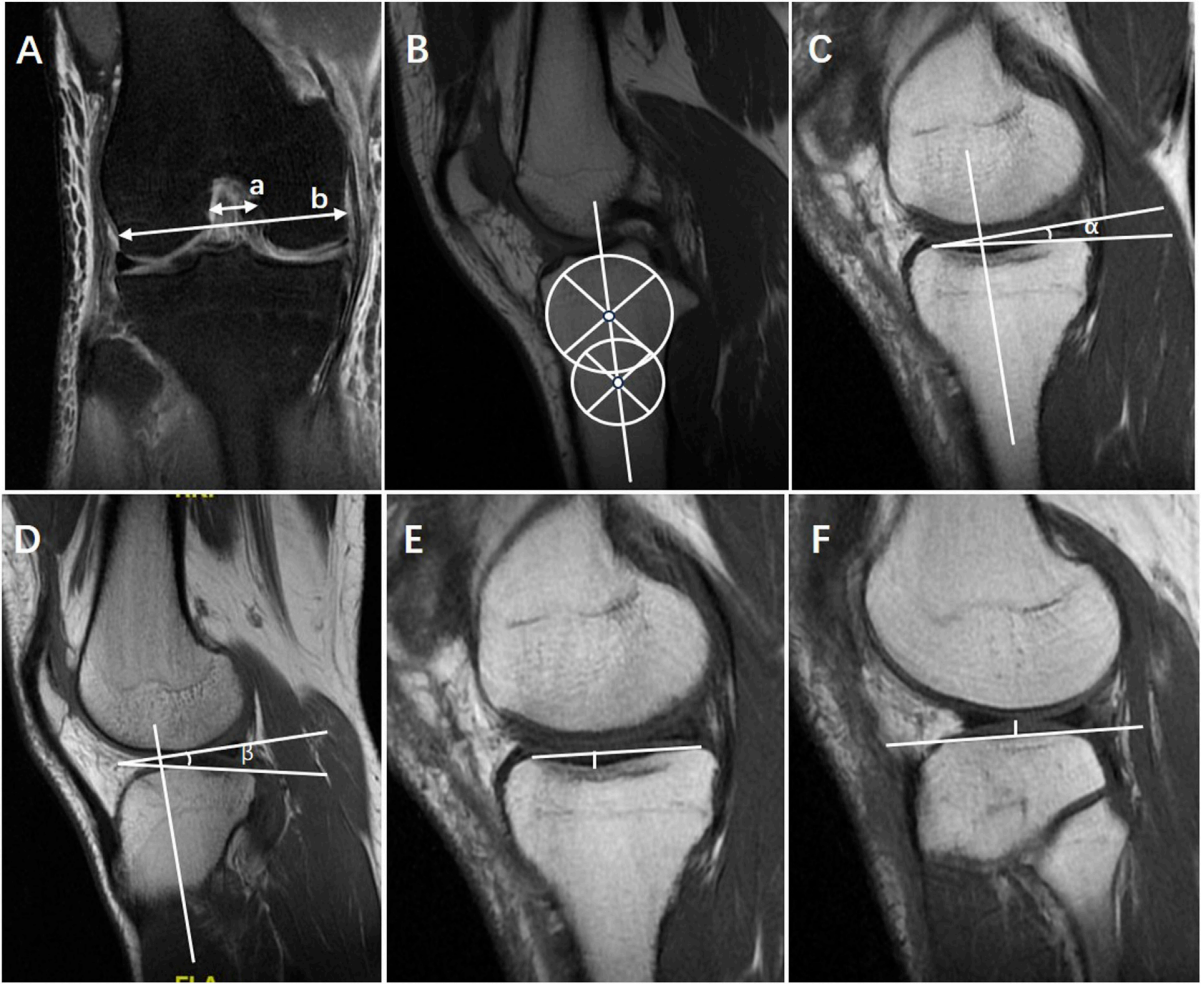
Other existing bone morphological risk factors were also measured by MRI with reference to the previous description, including (A) notch width index (NWI) = a/b, (B) the central axis of proximal tibia, (C) medial tibial slope (MTS) = α, on the center of the medial tibial plateau with the preserved longitudinal axis determined on the central sagittal slice, the tangent to the medial plateau was drawn to the cortex border, (D) lateral tibial slope (LTS) = β, on the center of the lateral tibial plateau with the preserved longitudinal axis determined on the central sagittal slice, the tangent to the lateral plateau was drawn to the cortex border, (E) medial tibial depth (MTD), (F) lateral tibial height (LTH).
